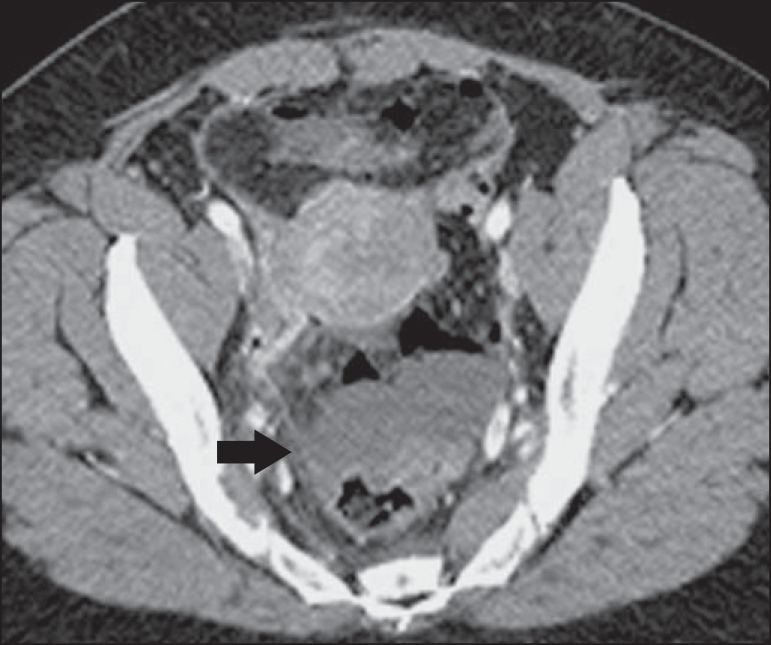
Intravenous contrast-enhanced axial CT of the abdomen showing
